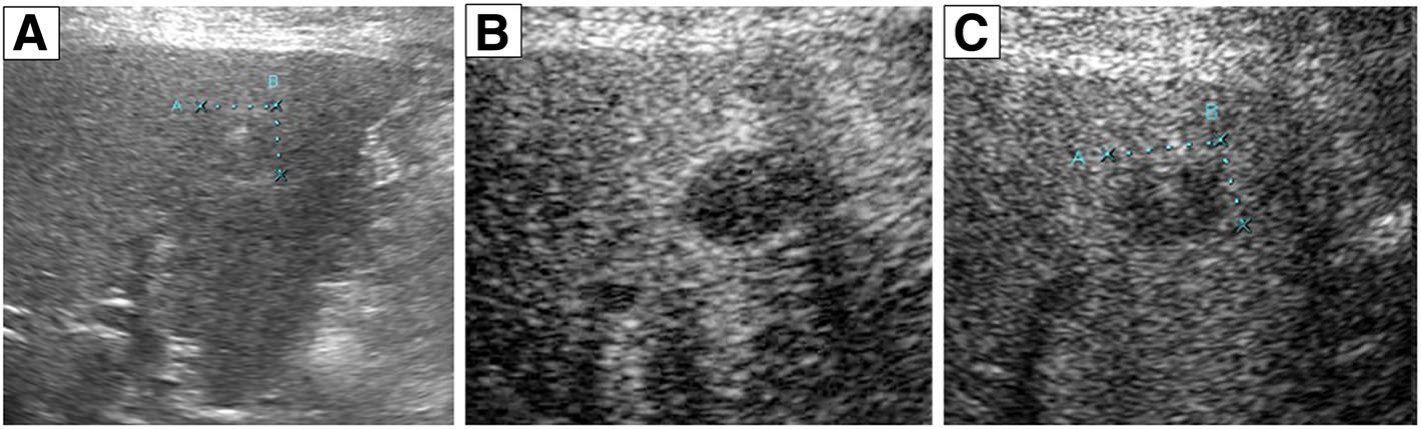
a: Ultrasonography showed intrahepatic bile duct dilatation and a low echoic lesion in S5. b,c: CEUS in the Vascular phase (b) and Kupffer phase (c) showed no enhancement
